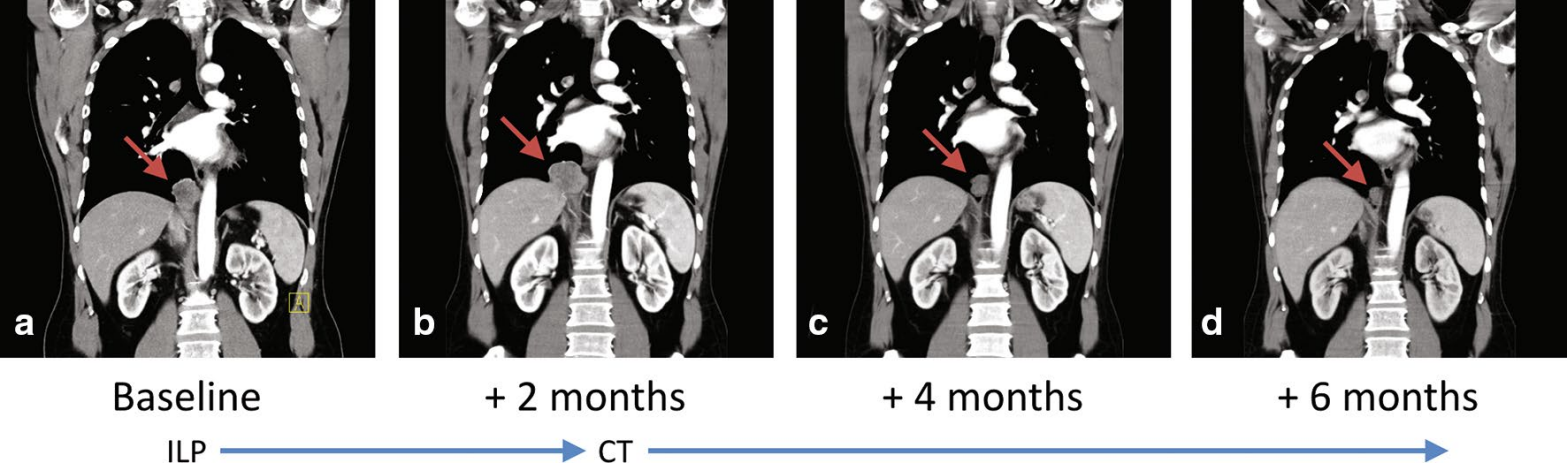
Coronal contrast enhanced CT scan. A right lung lower lobe metastases (arrow) at baseline (a), increasing after ILP (b), and progressively reducing after 3 (b) and 6 (d) cycles of chemotherapy with epirubicin and ifosfamide
